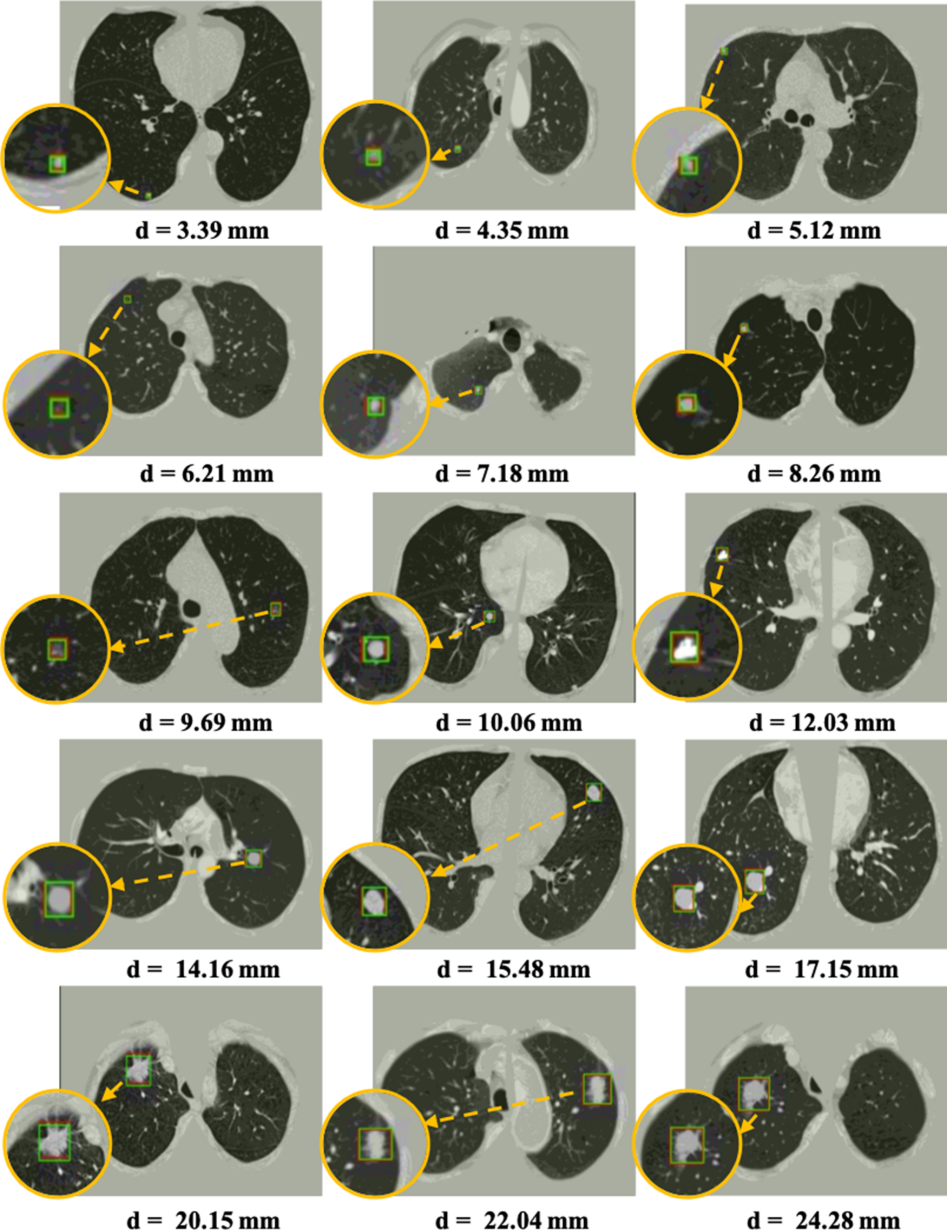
Visualization of some detected true nodules with different sizes from 3 mm to 25 mm in diameter d by our proposed *3DFPN-HS${}^{2}$* framework. For better visualization, the detected nodule regions are zoomed in, as shown in the orange circles. The green box indicates the predicted region, and the red box represents the ground-truth. Some of the red boxes are not observed because they are perfectly overlapped with the green boxes. The results demonstrate that our *3DFPN-HS${}^{2}$* framework is capable of detecting lung nodules of different sizes from CT scans accurately.
